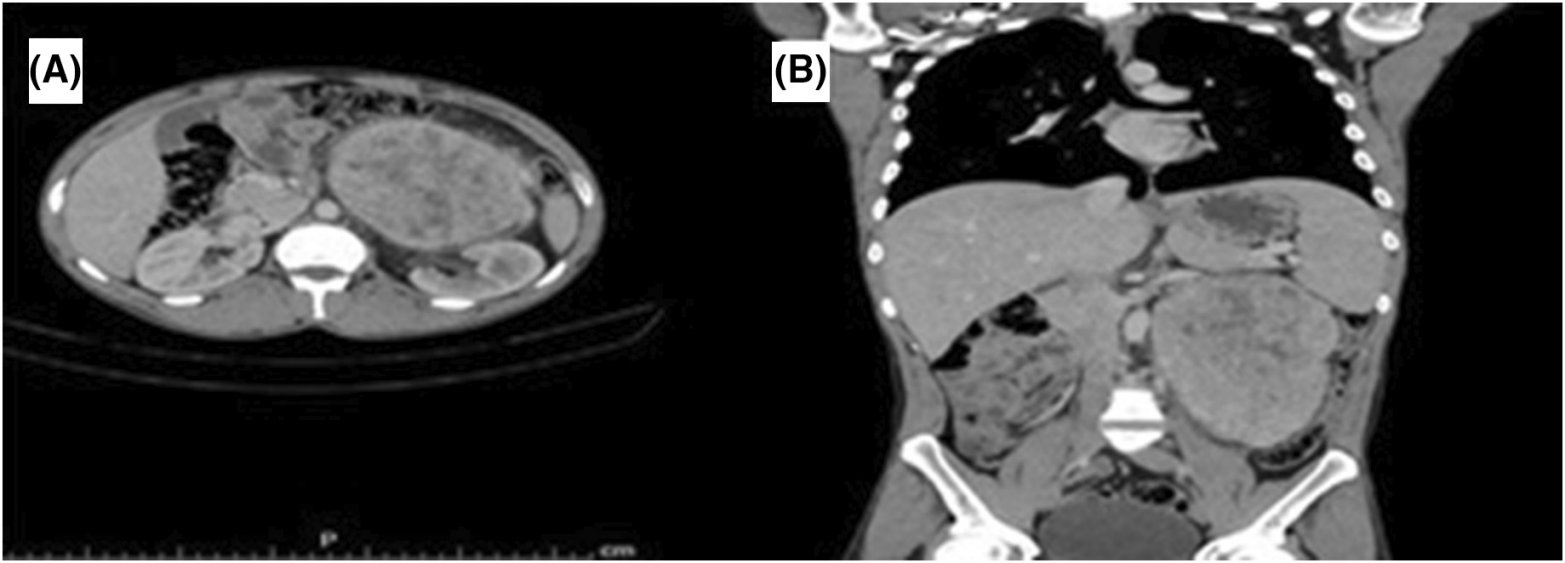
A CT scan picture showing the retroperitoneal mass.
